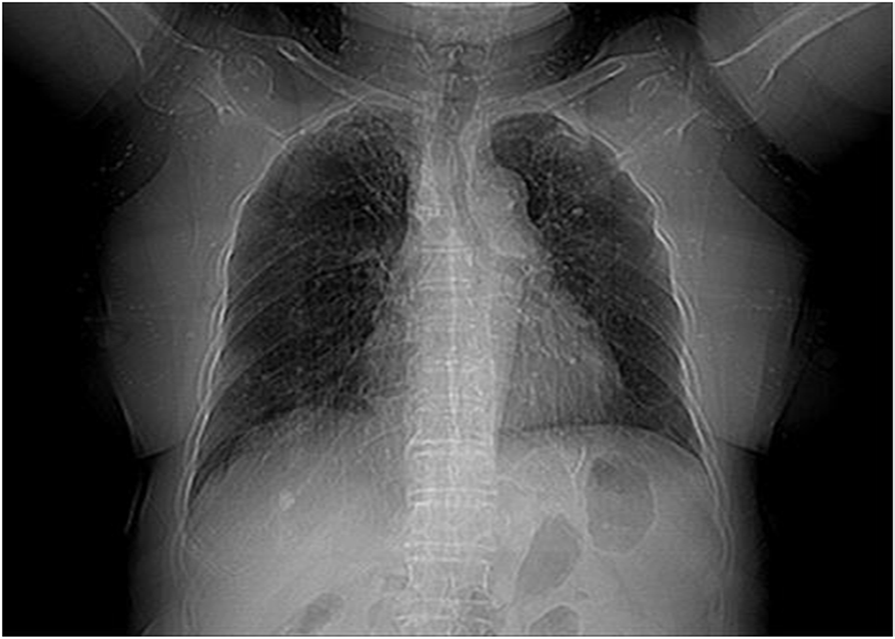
Sample of chest X-ray image of a Covid-19 patient
